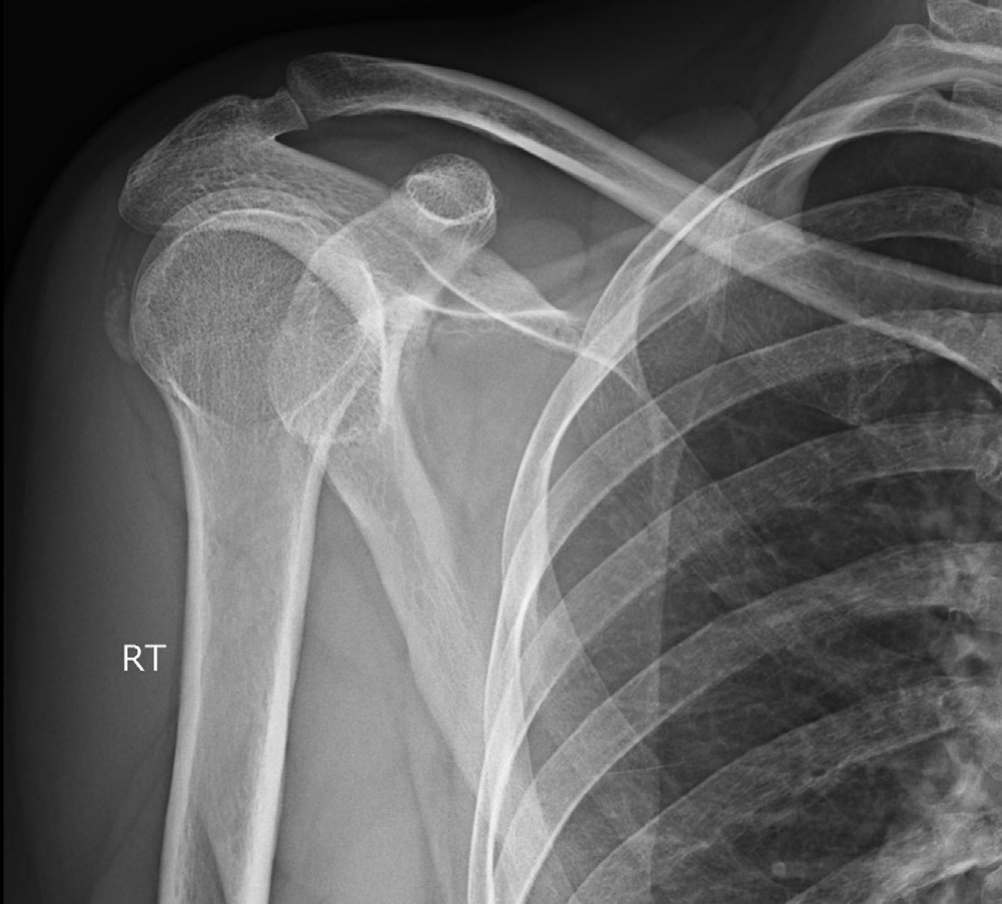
X-ray of a 41-year-old female patient who works as secretary, suffering from calcified tendinopathy before the start of the treatment protocol. The calcific deposit lies on the insertion of supraspinatus.
